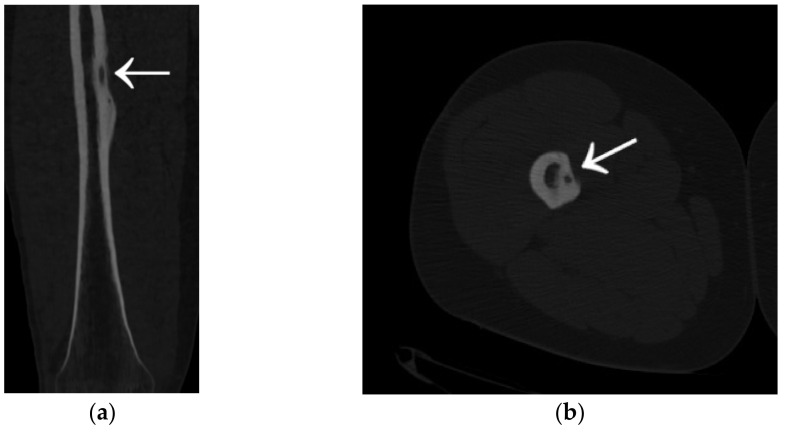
CT scans of the right femur showing flat cortical bone (arrows) after attempted resection and persistent focus of OO. (a) Frontal CT scan; (b) Transverse CT scan.
